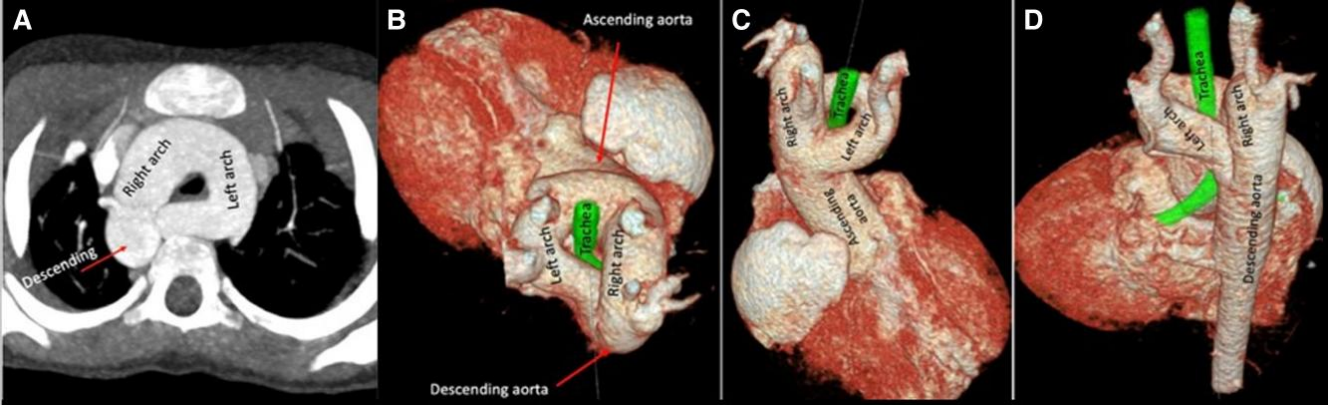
(*A*) Axial oblique reformatted maximum intensity projection images from cardiac computed tomography angiography provide diagnosis of a complete vascular ring made up of a right (dominant) and left aortic arches. This anomaly is the result of persistence of both aortic arches without regression of any segment. (*B*, *C*, *D*) Three-dimensional volume-rendered images in superior, anterior, and posterior views show trachea and oesophagus fully surrounded by two patent aortic arches.
